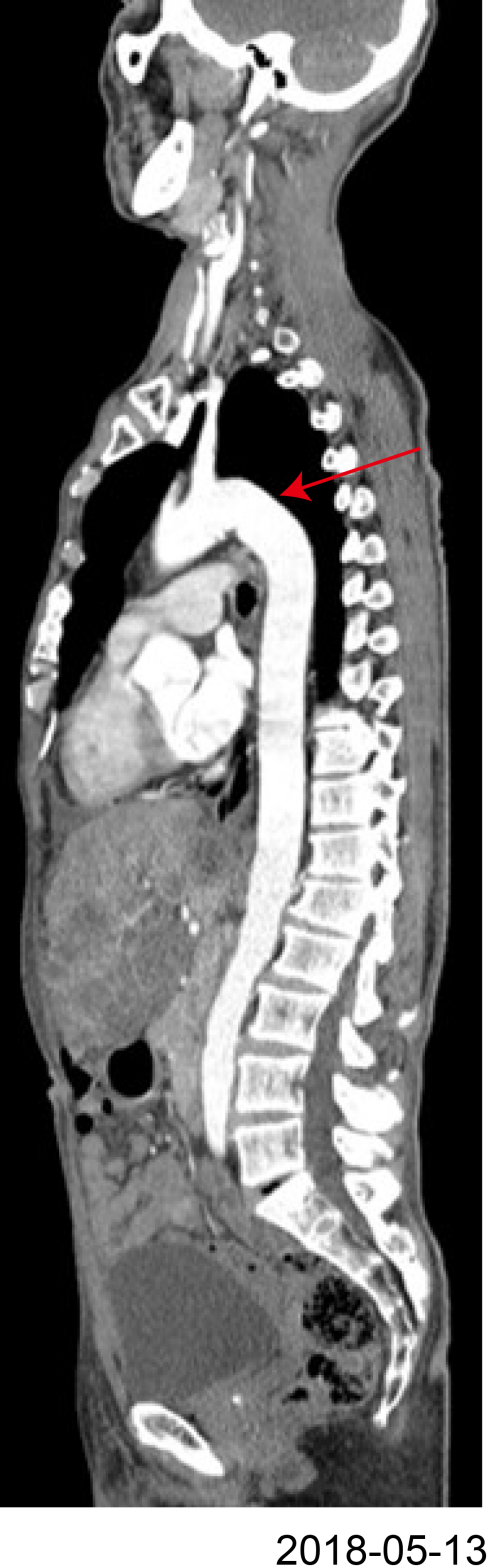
Computed tomography of thoracic aorta without aortic dissection before treatment.
